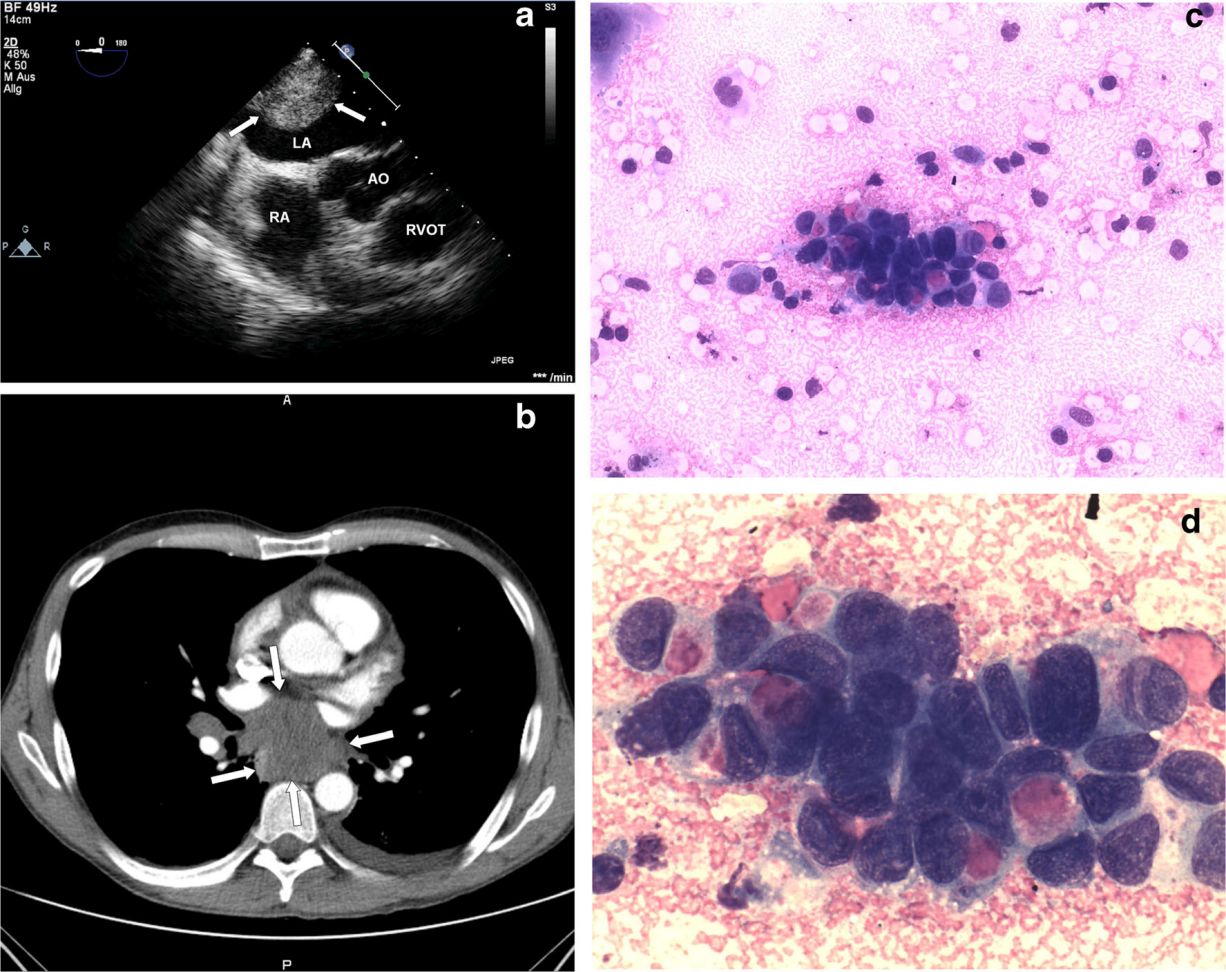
Transoesophageal echocardiogram (a) showing a left atrial mass (*arrows*). CT scan of the chest shows a polycyclic infracarinal tumour (*arrows*) with enlarged hilar lymph nodes and bilateral pleural effusion (b). An adenoid cystic bronchial carcinoma characteristically containing small, bland neoplastic cells and extracellular hyaline material was confirmed pathologically (c, d)
